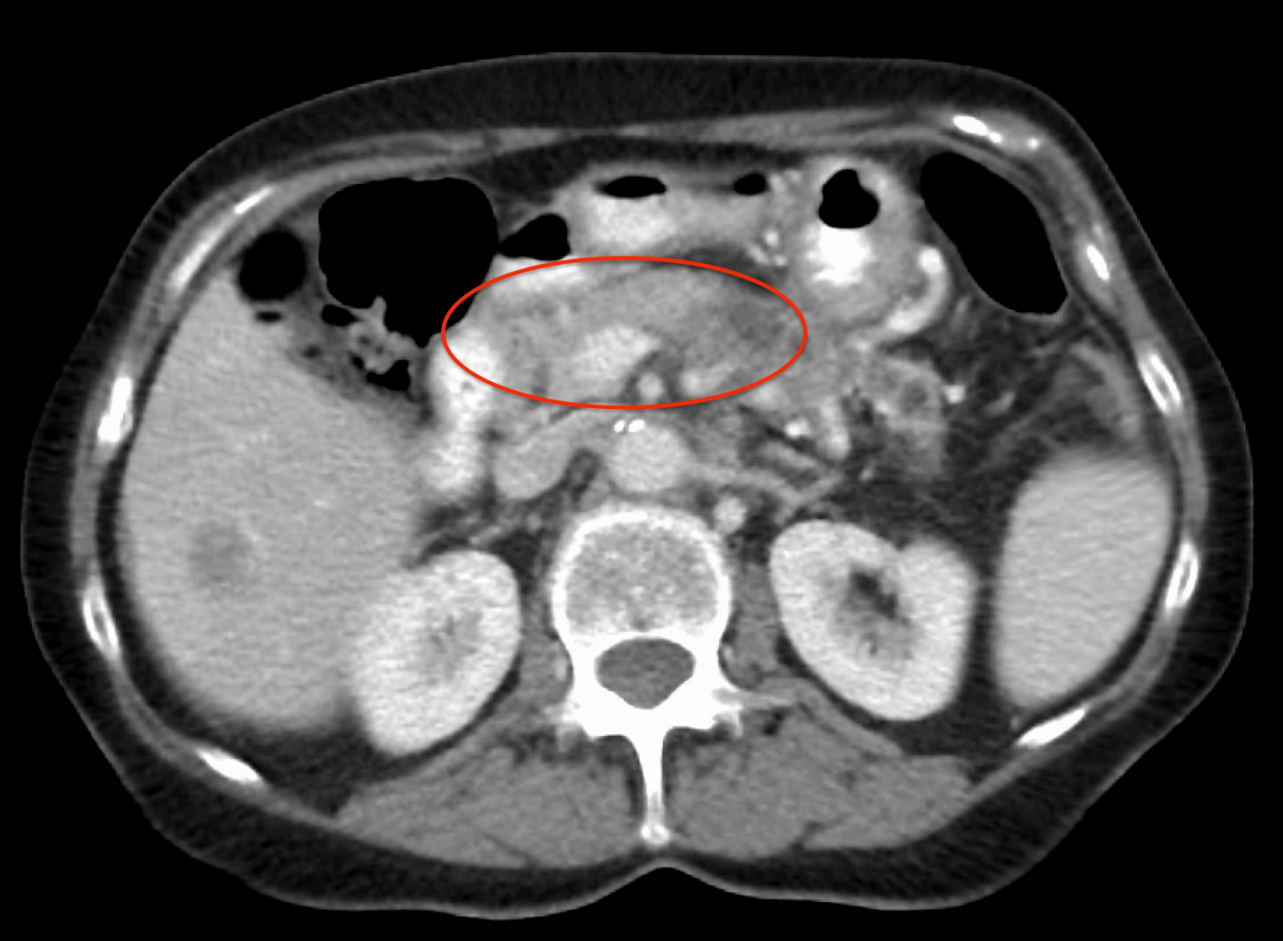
CT of abdominal and pelvis, revealing pancreatic mass
